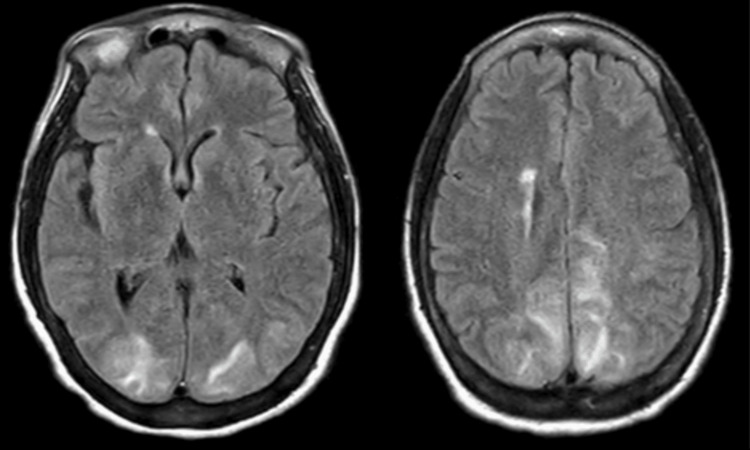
FLAIR MRI demonstrating diffuse gyral signal brightening bilaterally, most apparent posteriorly. Diffusion-weighted imaging (not shown) was negative for acute cerebral infarction. FLAIR: fluid attenuated inversion recovery
